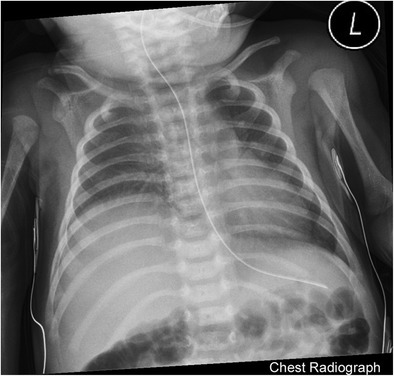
Neonatal Phrenic Nerve Palsy Chest radiograph of a 3-week-old with history of shoulder dystocia shows elevated right dome of diaphragm relative to left. Subsequently performed real-time ultrasound of the diaphragm (not included) revealed diminished excursion of the right hemidiaphragm relative to the left
